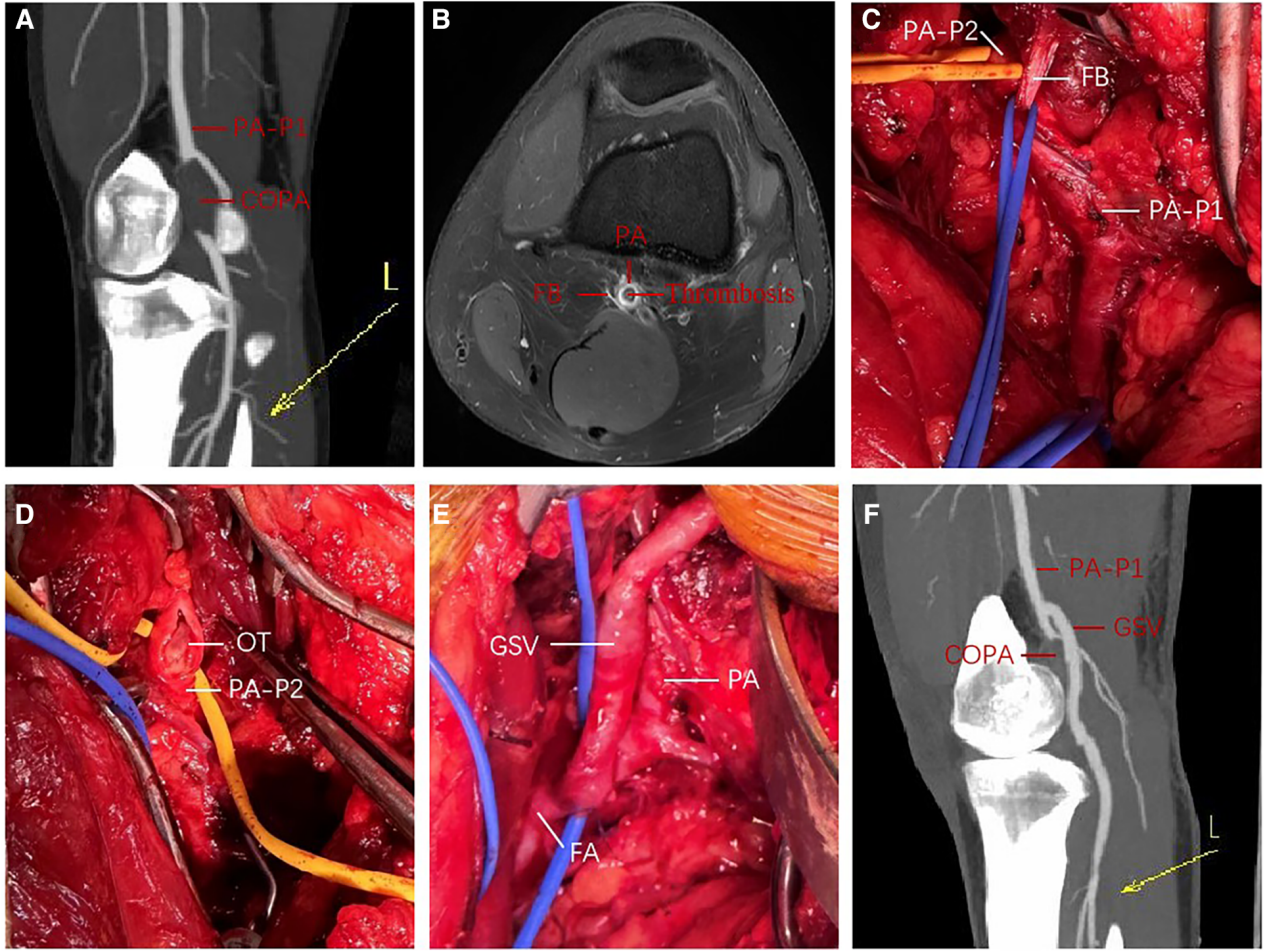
Perioperative findings in a young man with PAES; (A) preoperative 3D contrast-enhanced CTA of left lower extremity; (B) preoperative enhanced MRI T1W imaging of left knee joint; (C,E) intraoperative exploration and left popliteal artery autologous saphenous vein bypass; (F) 3D contrast-enhanced CTA of left lower extremity at 3 months after operation. COPA, completely occluded popliteal artery; CTA, computed tomography angiography; FA, femoral artery; FB, fibrous band; GSV, great saphenous vein; L, left lower extremity; MRI, magnetic resonance imaging; OT, organic thrombus; PA, popliteal artery; PA-P1, the P1 segment of the popliteal artery; PA-P2, the P2 segment of the popliteal artery; PAES, popliteal artery entrapment syndrome; 3D, three-dimensional.
